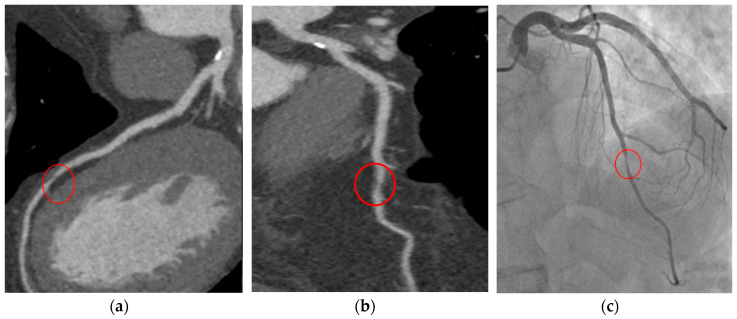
T.F. 67 years-old, male; dyslipidaemia, smoker; typical exertional chest pain; exercise test negative; CCTA (a,b): subocclusive stenosis of mid-LAD; ICA (c): significant stenosis was confirmed and elective PCI was performed. CCTA: coronary computed tomography angiography; LAD: left anterior descending artery; ICA: invasive coronary angiography; PCI: percutaneous coronary intervention.
